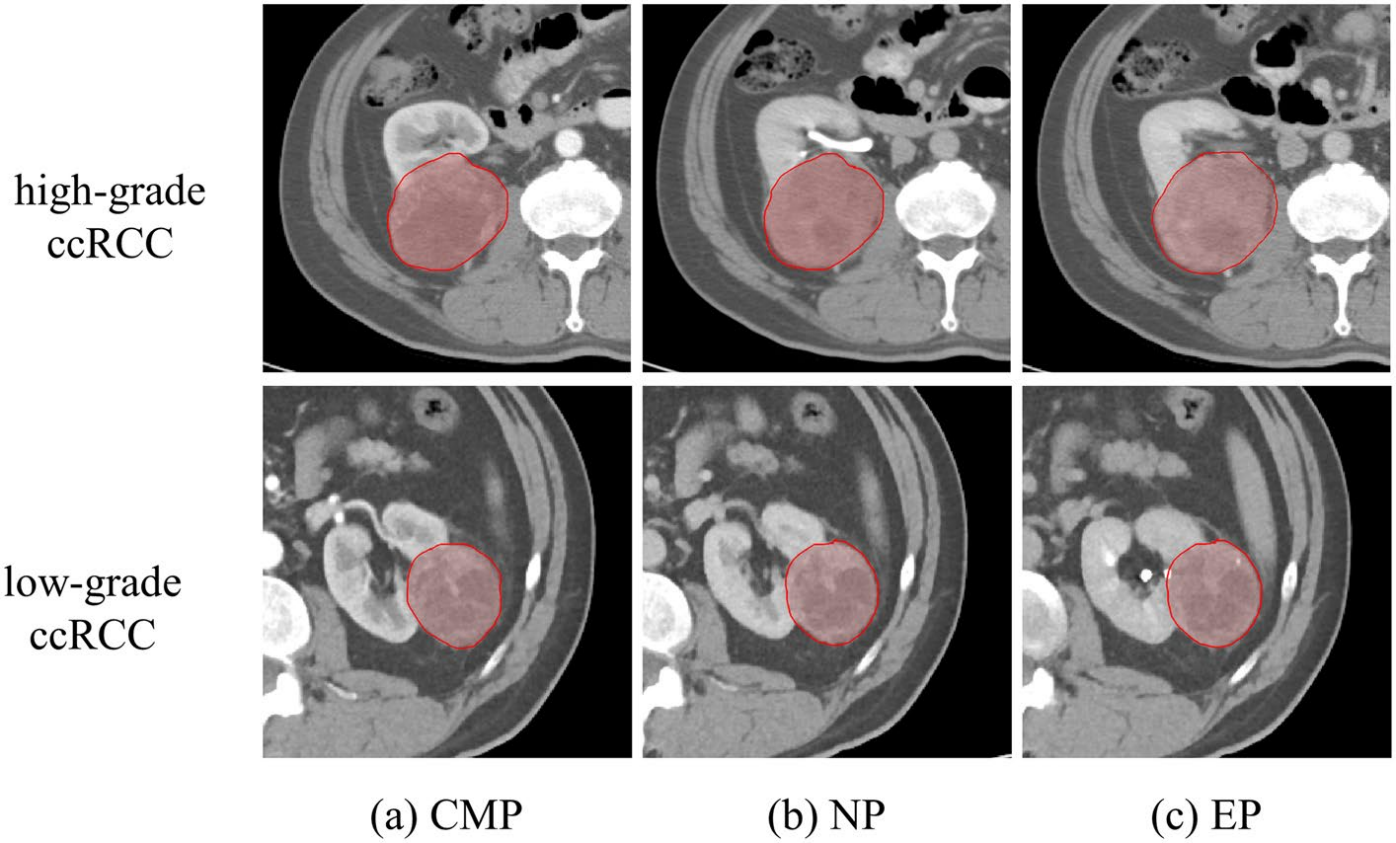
An example of the manual segmentation on multiphase CT of high-grade and low-grade ccRCC. (a–c) show the tumor regions of CMP, NP, and EP. Everyone also shows the ROI regions drawn based on the CT images.
